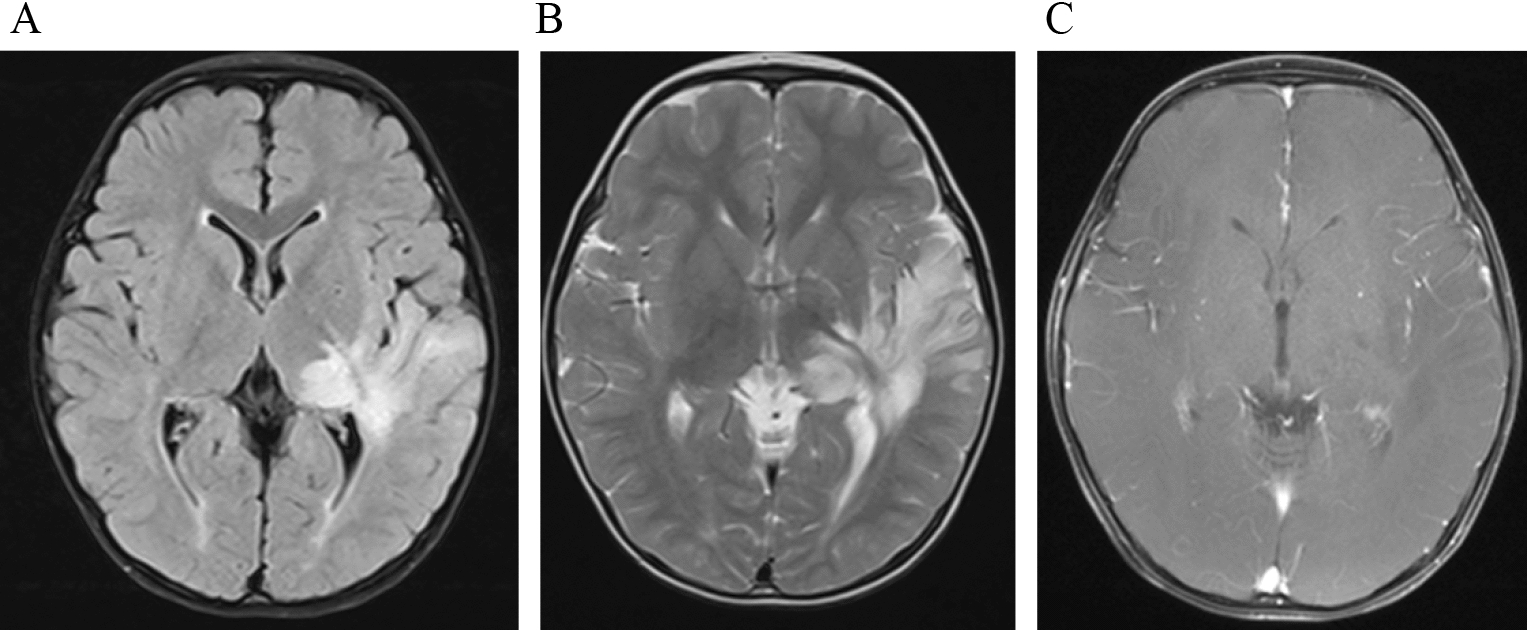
Imaging findings of pediatric patients with autoimmune GFAP astrocytopathy and with positive MOG antibodies. The abnormal hyperintensity lesions on T2-weighted (A) and FLAIR images (B) were observed in unilateral basal ganglia, hypothalamus and temporal lobe and without abnormal enhancement
